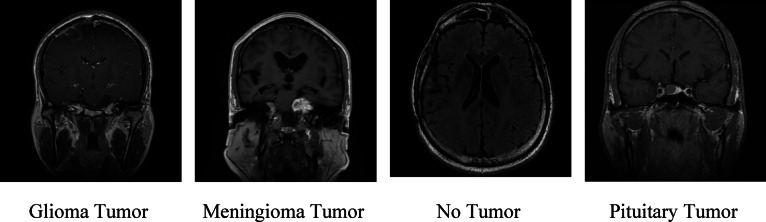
Brain MRI scans classifying the tumor^15^.
